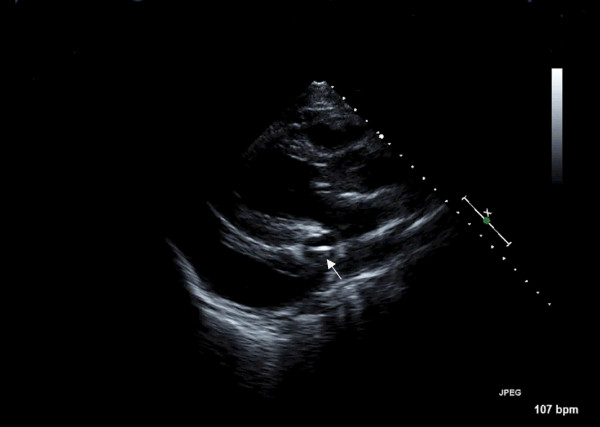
Echocardiogram demonstrating the Swan-Ganz catheter (seen in cross section and indicated by the small arrow) passing via dilated coronary sinus.
